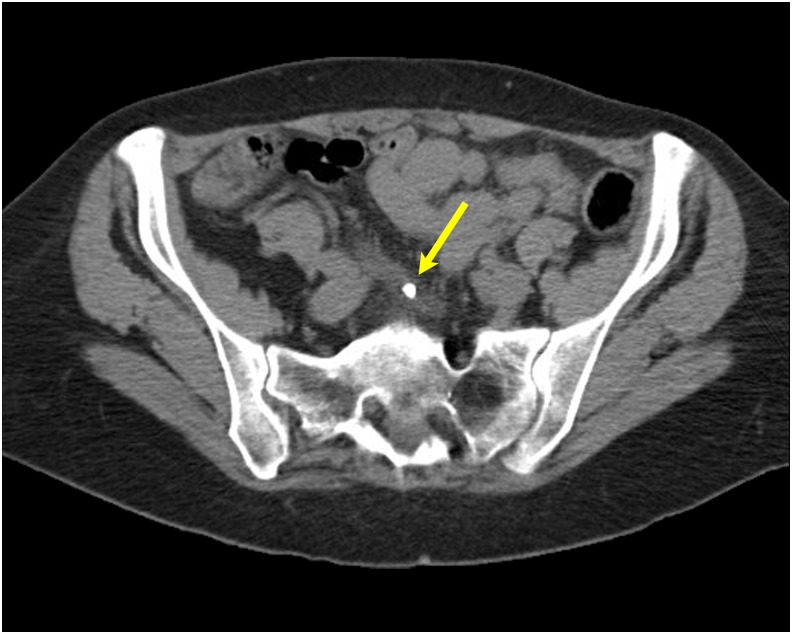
CT scan with stone in the mid ureter shown by the *yellow arrow*. CT, computed tomography.
